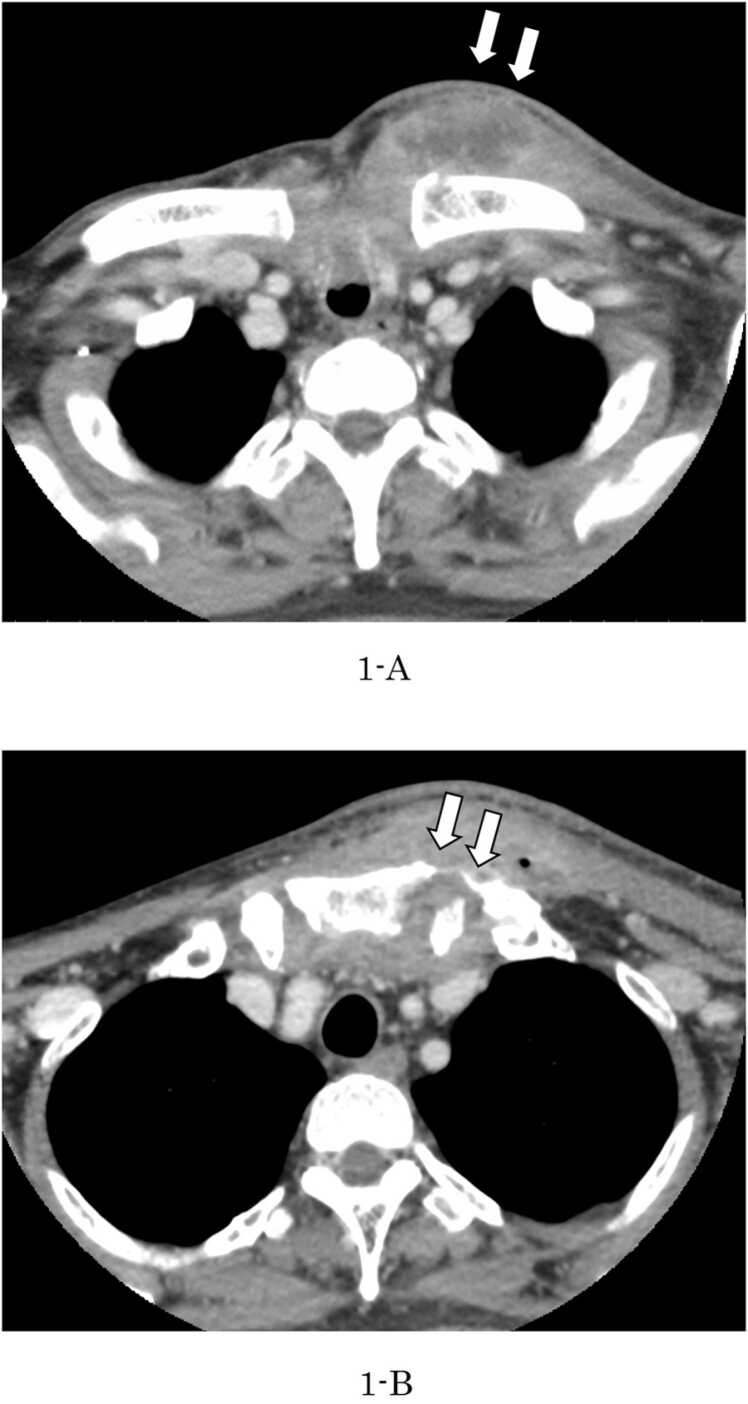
Computed tomography showed abscess formation around left clavicle (A), inside the sternum and an osteolytic region was observed on the left side of the sternal stalk (B), as indicated by the arrow.
